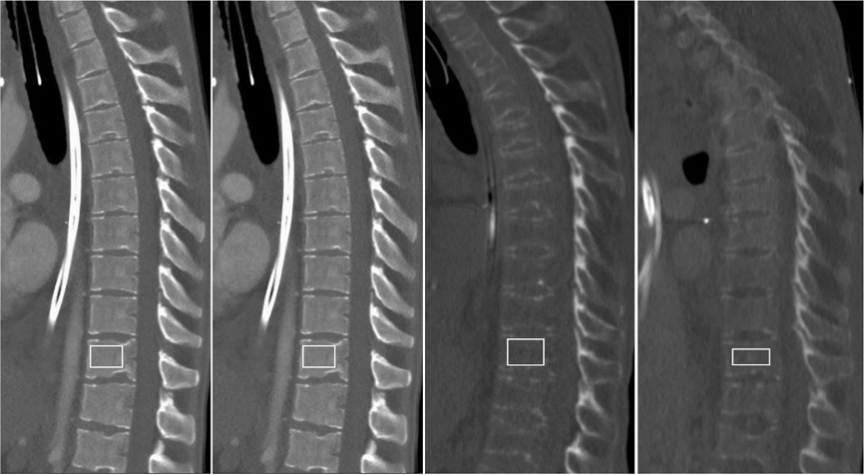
Sagittal plain computed tomographic imaging of the vertebral column over time. Computed tomographic imaging from four different time points are shown. Region of interest within the thoracolumbar vertebral body is marked with a white square. Corresponding Hounsfield Units (HU) at 3 years prior admission (242 HU), admission (238 HU), day 95 of CVVHD (52 HU), and day 226 of CVVHD (90 HU) are labelled accordingly. Three years prior to admission bone mineral density (BMD) was within physiological limits with age-adjusted configuration within normal limits for the thoracolumbar vertebrae. No significant change was found at admission. A significant decrease in BMD was noted at 4.5 months after admission (day 95 of CVVHD), with radiodensity decreasing to 52 HU preventing differentiation between the vertebral body edge and the spinal canal. BMD increased to 90 HU after intervention.
